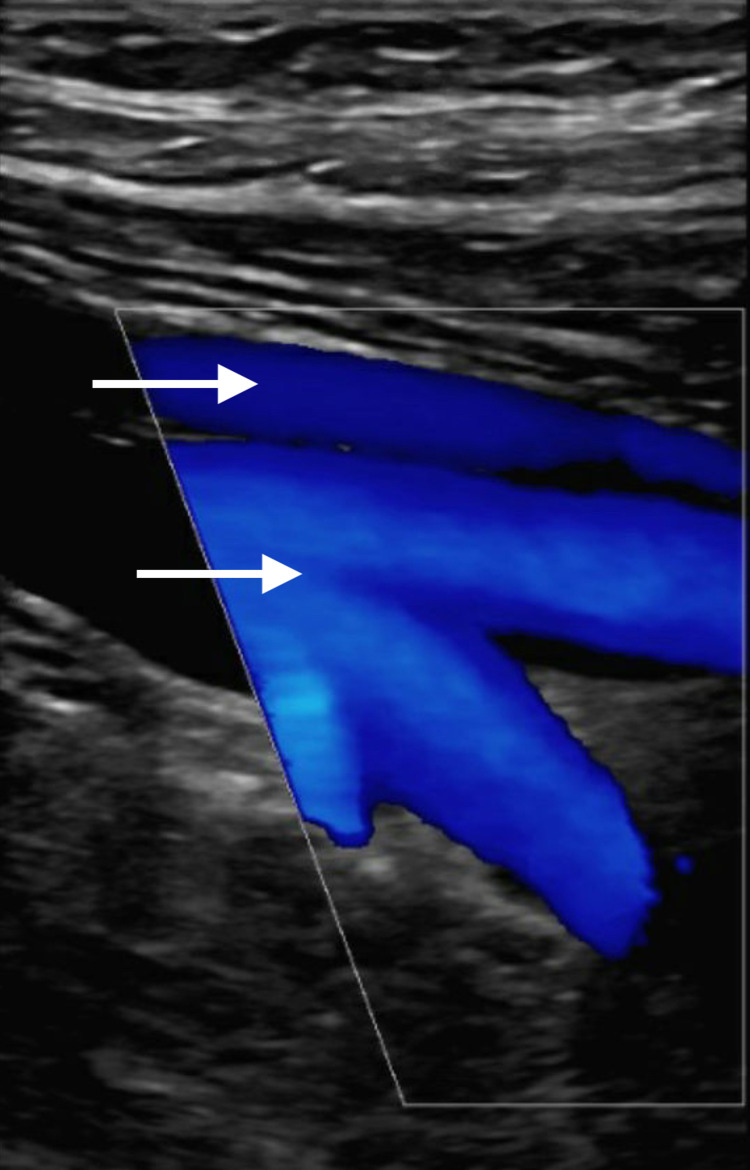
Ultrasonography of right proximal superficial and deep femoral vein reveals normal vasculature.
